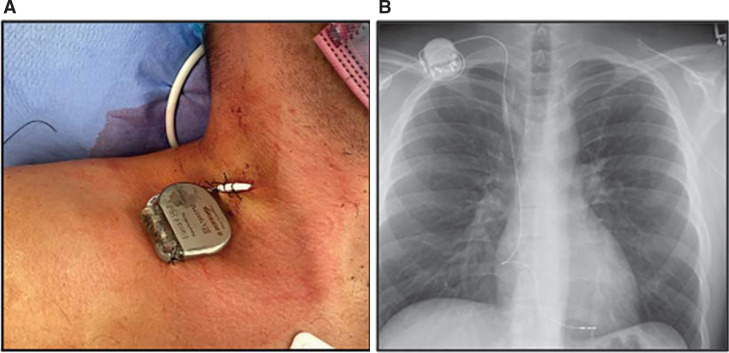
A single-chamber temporary permanent pacemaker. A: Implantation via the right internal jugular vein. B: Chest X-ray confirming apical placement of the right ventricular lead via the internal jugular vein.
